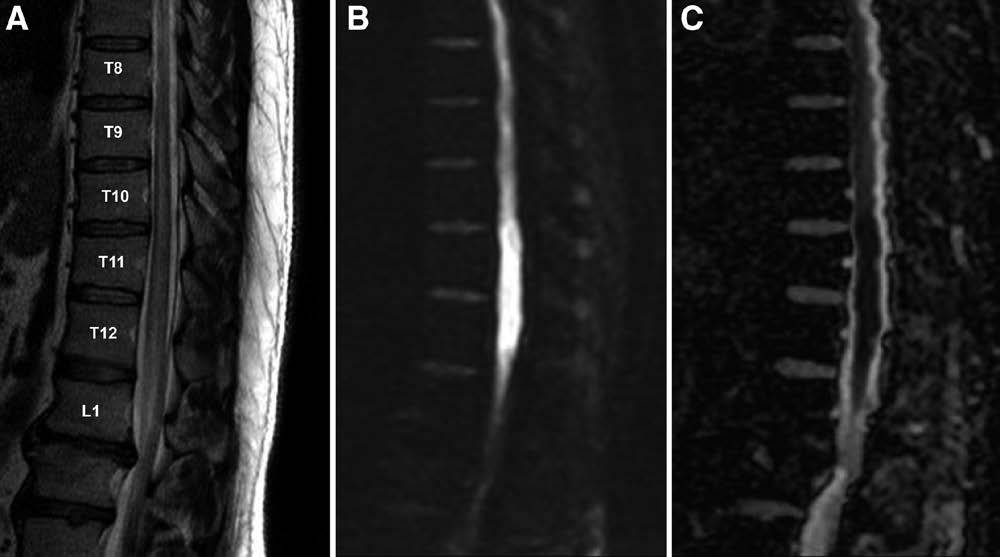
Sagittal magnetic resonance images. T2-weighted sequences show high signal intensity lesions within the central gray matter of the thoracic cord, extending from the T9 to L2 levels. (A) And high signal intensity on the sagittal diffusion-weighted image and low signal intensity on the apparent diffusion coefficient map demonstrates the acute phase of spinal cord infarction. (B and C).
